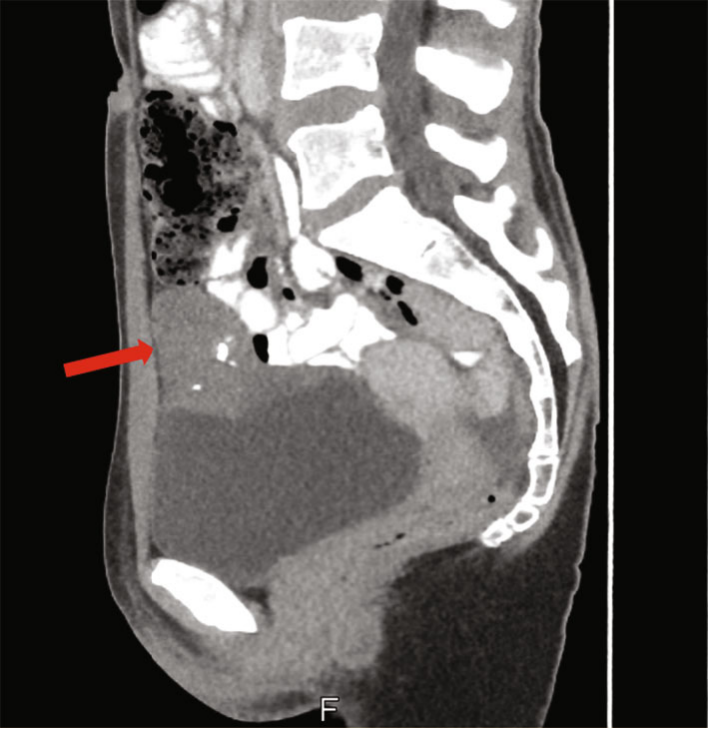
CT of the abdomen in the coronal plane, showing the tumorous mass on the urinary bladder dome with the presence of calcifications (red arrow).
